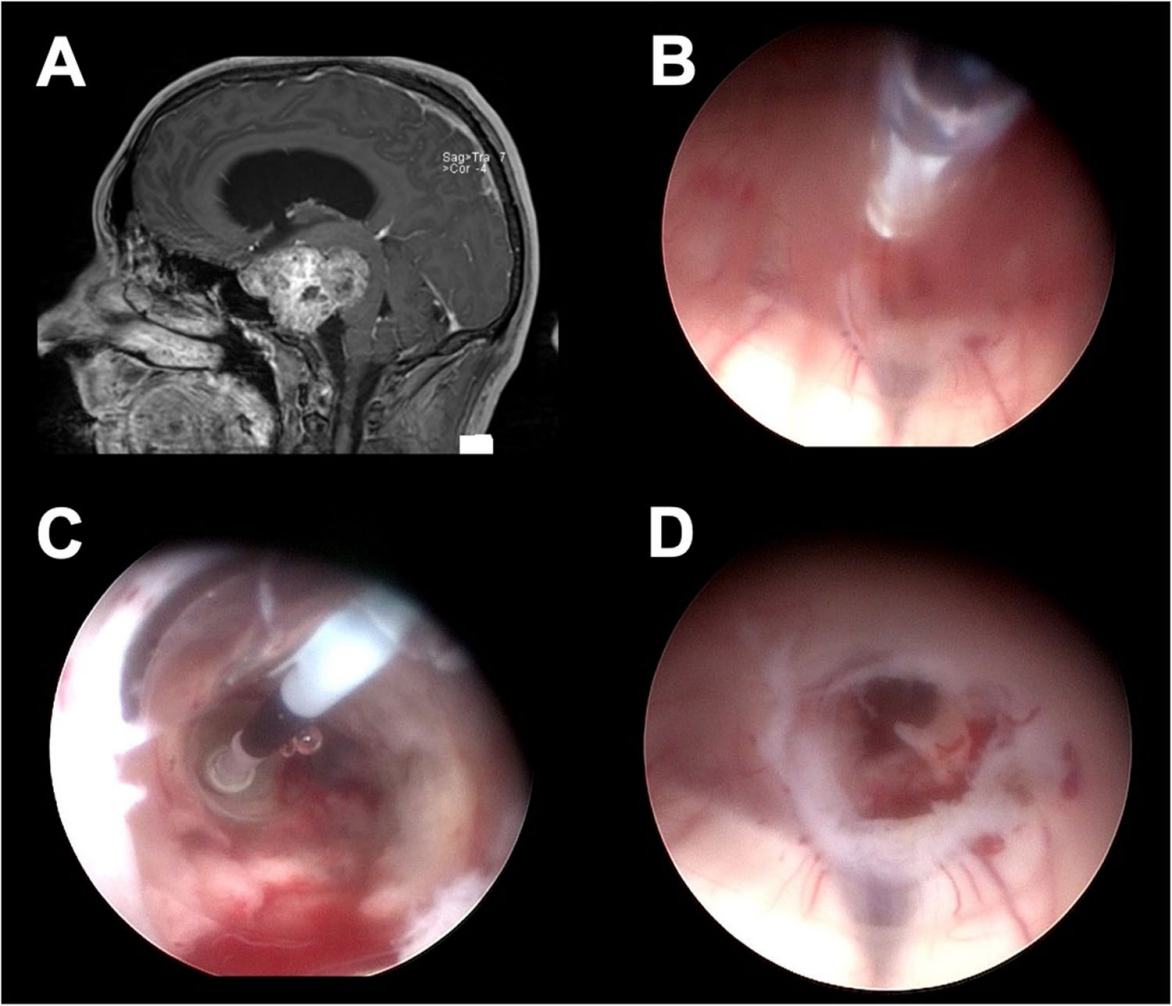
Example of the application of the compression technique. Preoperative MRI of a 42-year-old patient with clivus chordoma (A). The patient was scheduled for an ETV due to obstructive hydrocephalus and biopsy. A hemorrhage occurred at the stoma edge during the ETV (B). The balloon catheter was reintroduced and dilated for a short amount of time (C). The hemorrhage stopped and the surgeon was able to proceed with the tumor biopsy (D)
